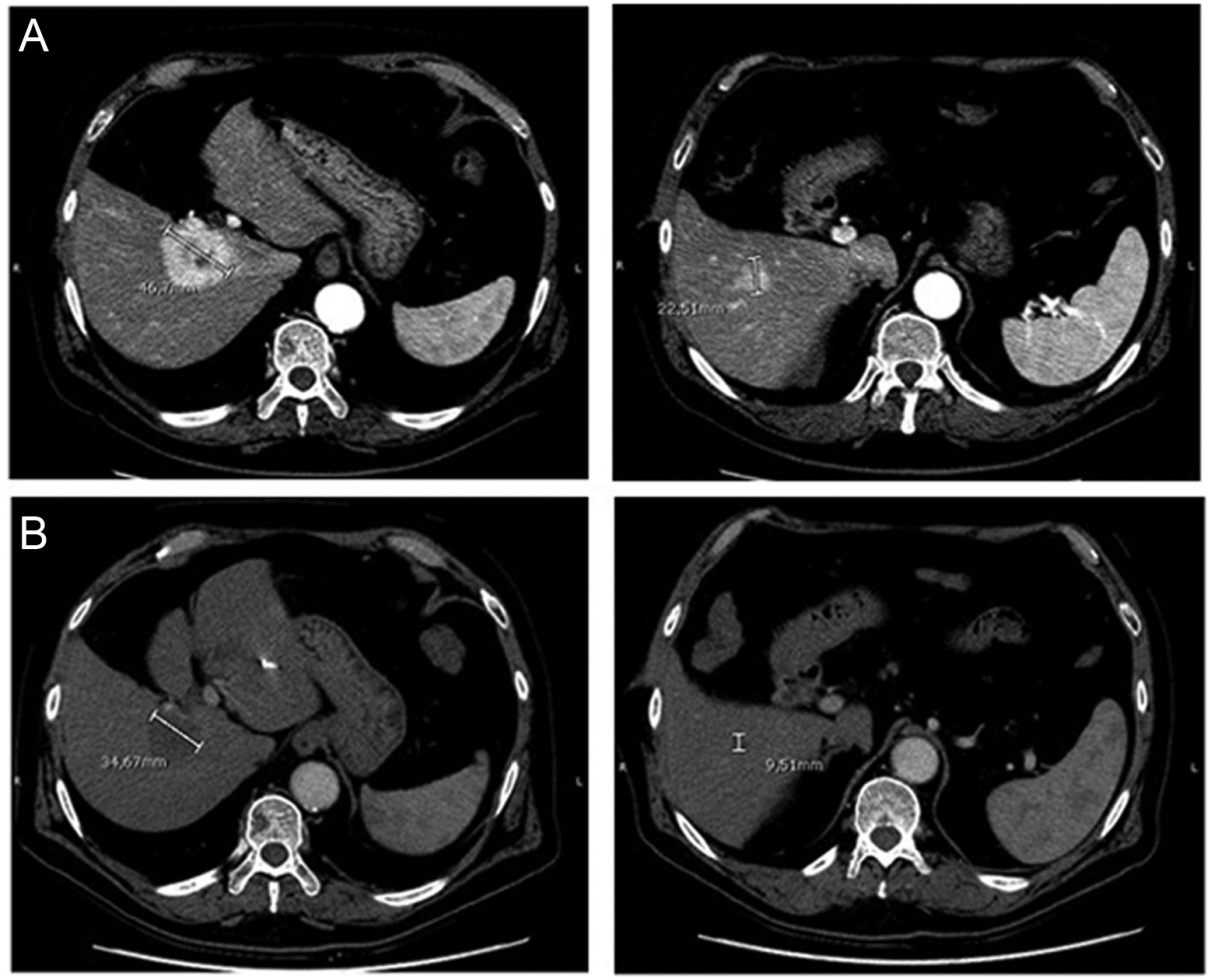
CT scan images of a patient with liver metastases of MTC in the right hepatic lobe before (A) and 1 month after TARE (B). CR was documented according to mRECIST.
